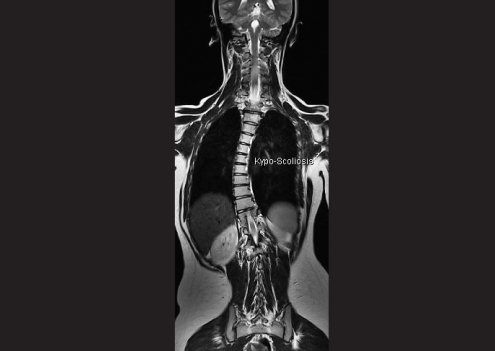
MRI spine of case 2, T2 coronal
